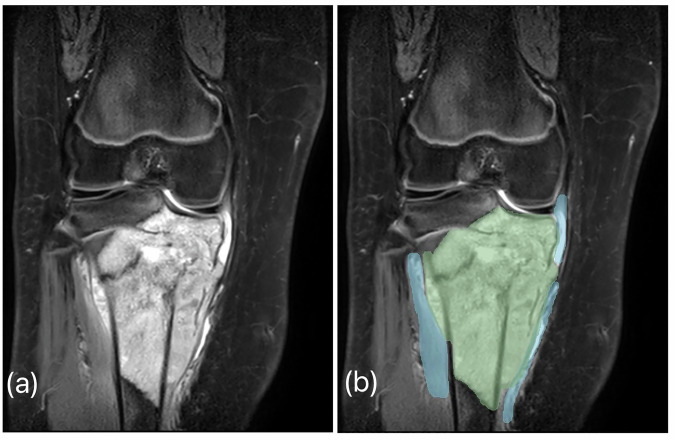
a Coronal fat-suppressed T2-weighted image shows an intramedullary lesion in meta-epiphysis of the proximal tibia with associated peritumoral soft tissue edema. b Tumor segmentation overlay on the original image, with the green area representing the tumor and the blue area indicating the peritumoral region
